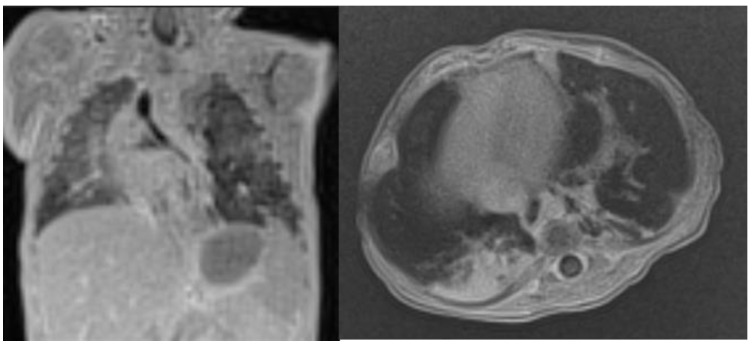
Lung MRI at 3 months of age: the mass of the left lung is barely detectable, and a slight mediastinal shift is still present.
